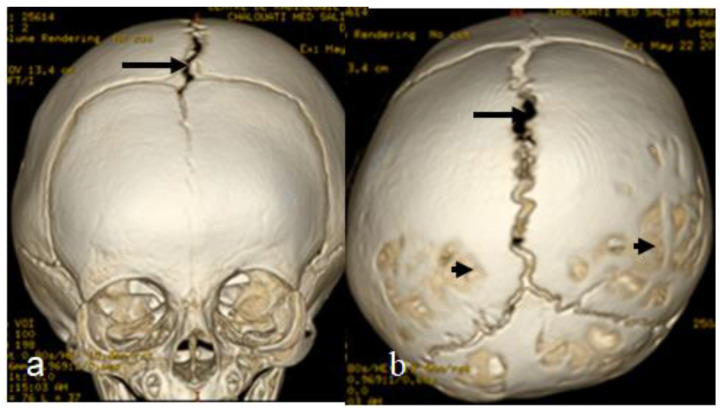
Three-dimensional reconstruction CT scan of the skull (anterior view) of a 2-year-old boy with RSS showed the frontal bossing and the persistent open anterior fontanelle-(arrow) (a); Three-dimensional reconstruction of the crown of the skull of the same boy showed defective ossification of the anterior fontanelle and defective and disorganized ossification of the skull bones (arrow heads) (b).
